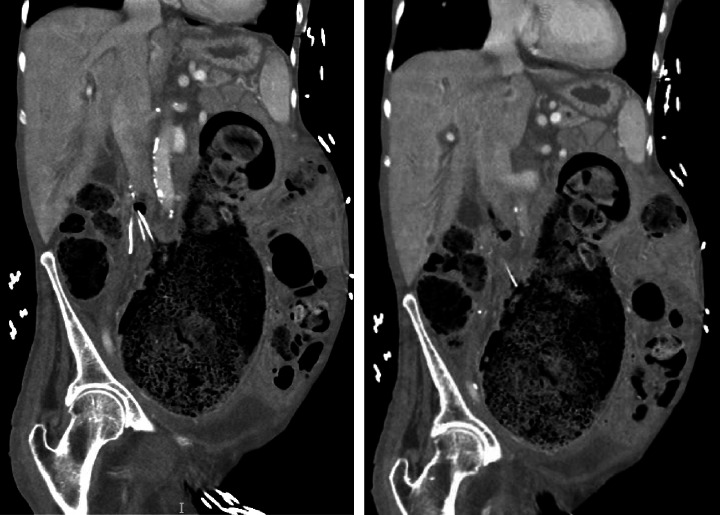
CT abdomen and pelvis coronal views. Large, dilated, and stool-filled sigmoid colon. Malpositioned IVC filter leg (long strut) penetrating the colon wall with associated intracaval air at the filter neck visible laterally.
